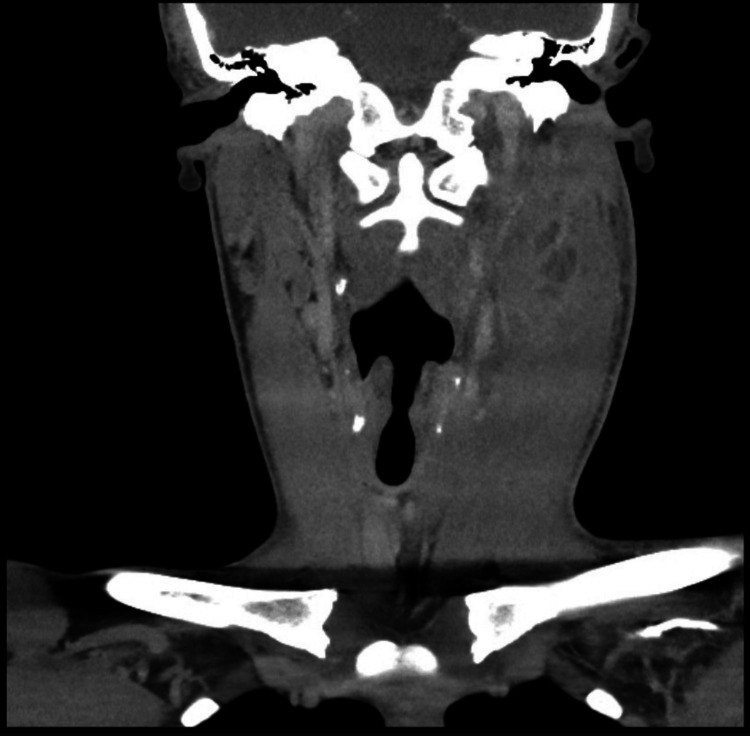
Coronal view of contrast-enhanced CT of the neck of a 19-year-old male immunocompetent athlete, demonstrating intra-muscular collection within the left SCM in the absence of local or regional source. SCM: Sternocleidomastoid muscle
